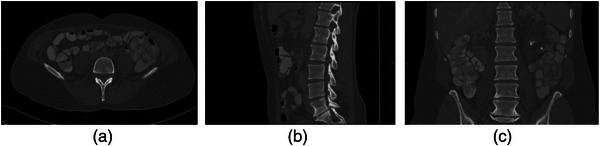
Example of computed tomography (CT) image of lumbar spine. (a) horizontal plane; (b) sagittal plane; (c) coronal plane.
